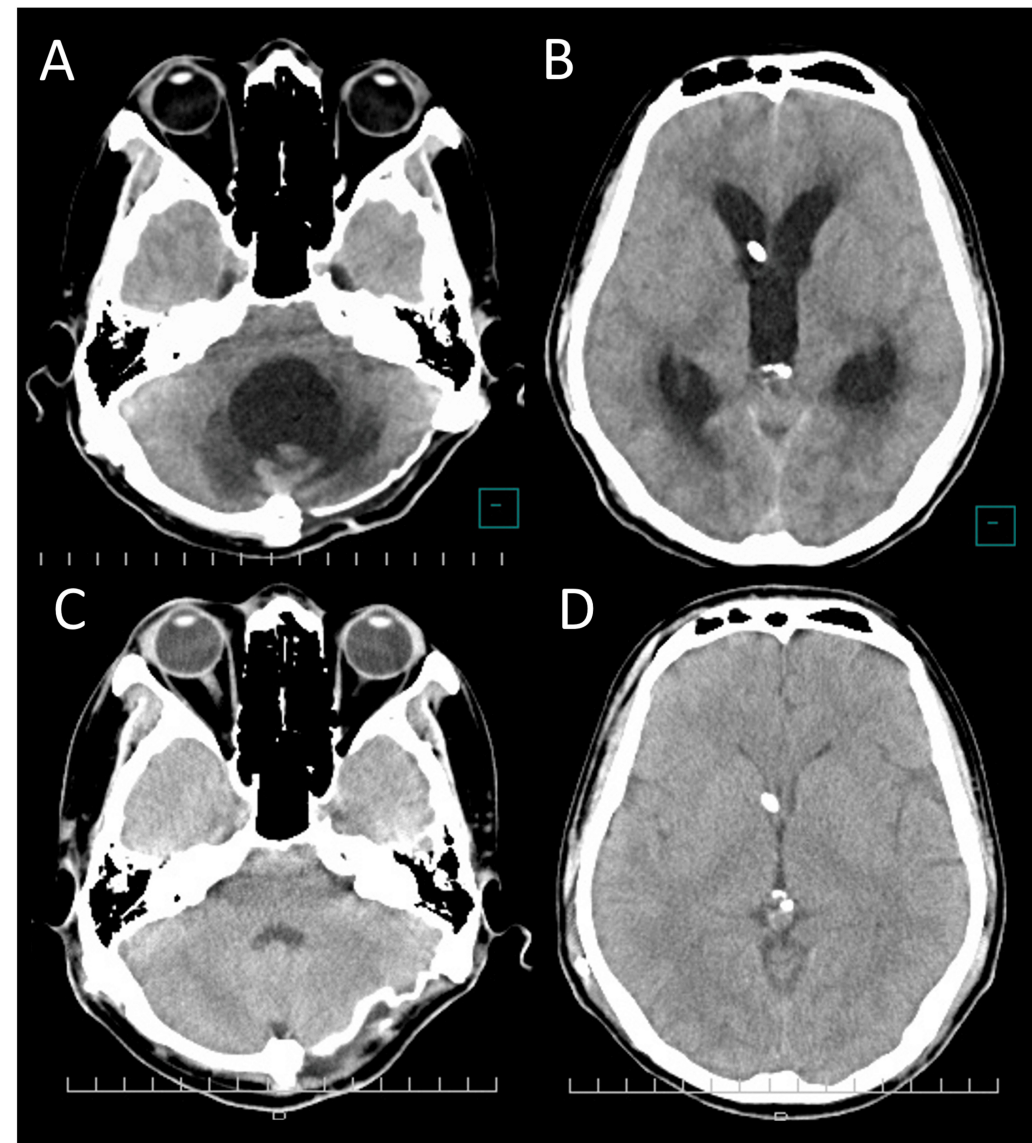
Serial CT scans during shunt valve adjustments. (A, B) Ventricular enlargement is present. (C, D) Ventricular size is reduced following shunt pressure adjustment, consistent with overdrainage.
